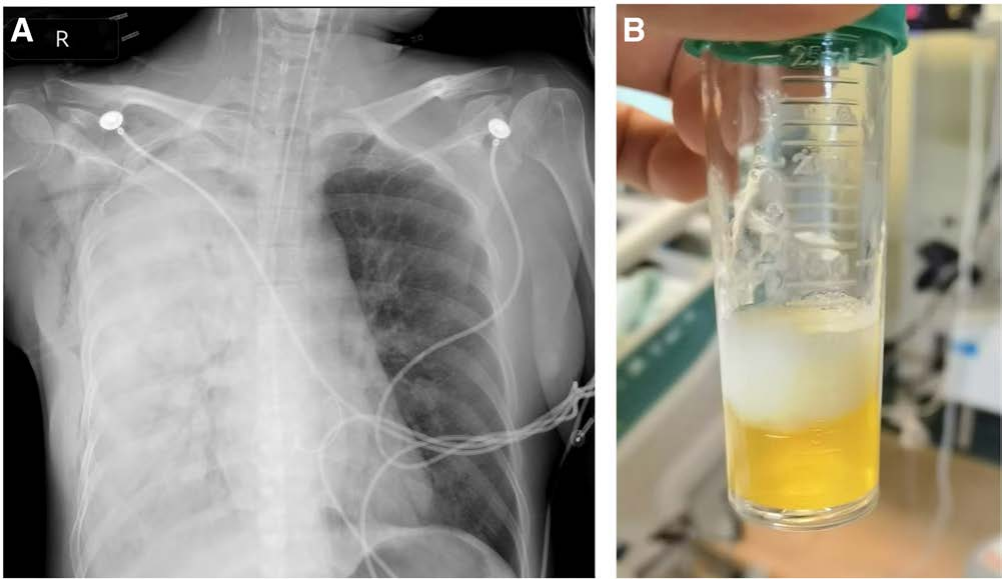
(A) Bedside chest radiograph showed significant lung consolidation on the right side corresponding to the surgical approach, but the left side was relatively normal. (B) Postoperative bronchoscopy showed a large amount of yellowish airway secretions from the right bronchus, which was removed by negative pressure suction.
